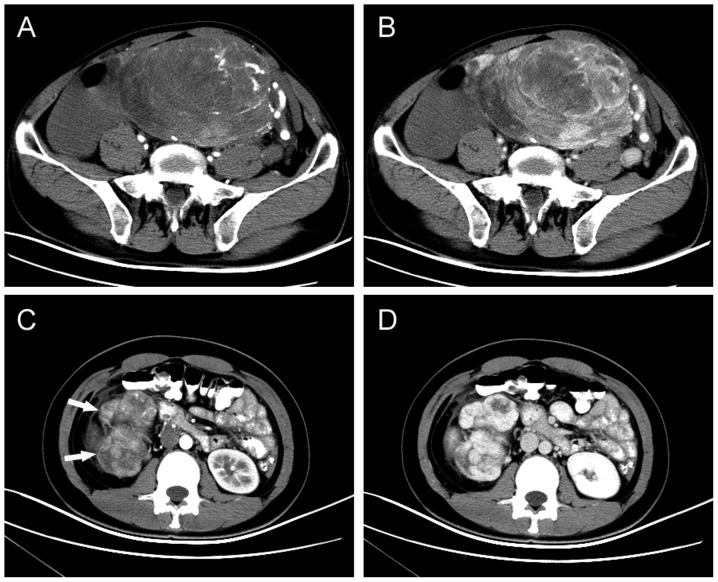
Typical imaging manifestations of abdominopelvic SFTs. (A) Arterial-phase and (B) venous-phase axial contrast-enhanced CT scan showing a well-defined, intensely enhancing mass in the pelvis with central nonenhancing areas. (C) Lobulated SFTs (indicated by arrows) of the retroperitoneum in a 21-year-old female. (D) Axial contrast-enhanced CT scan, obtained in the arterial phase, revealing a well-defined hypervascular mass with intense enhancement. SFT, solitary fibrous tumor; CT, computed tomography.
